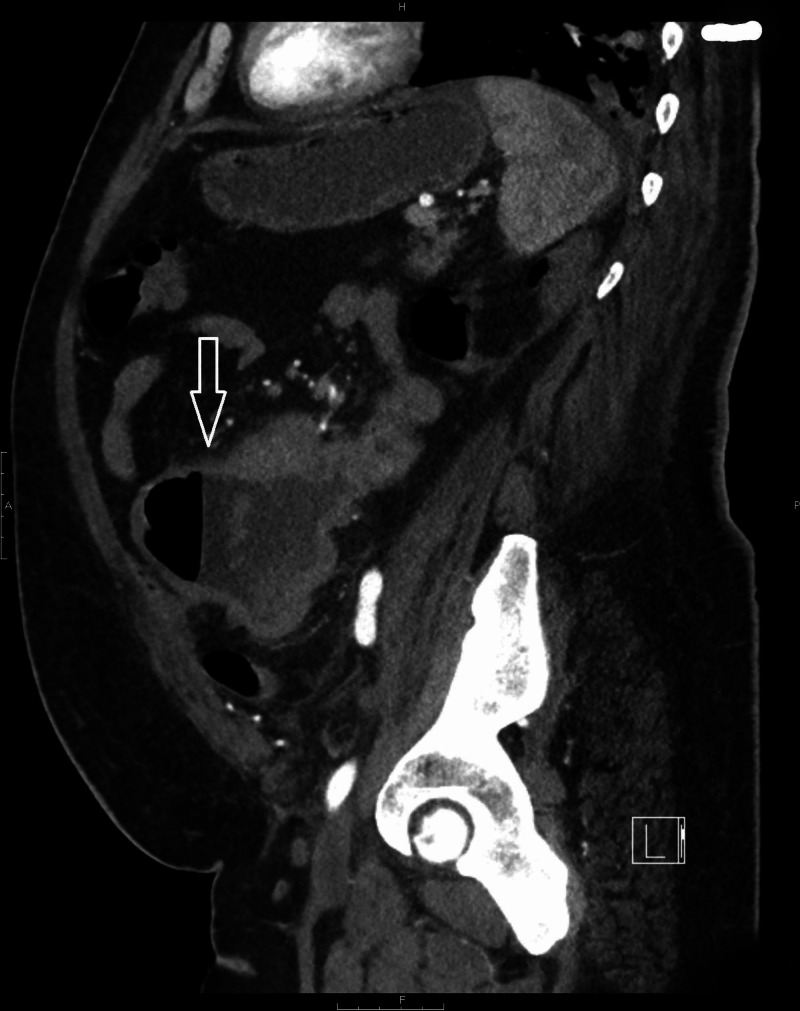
CT scan of the abdomen and pelvis: sagittal view
